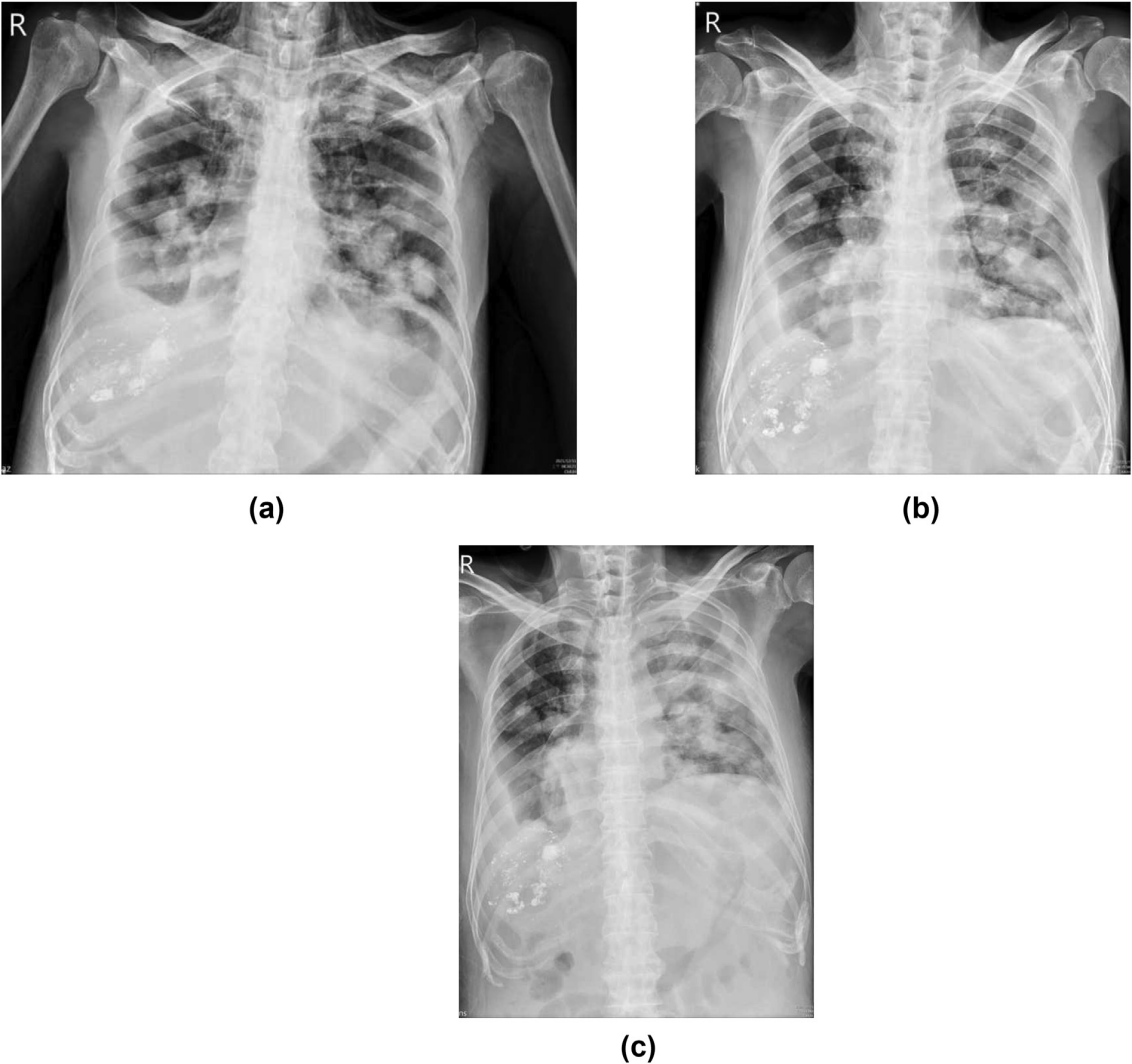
a. The anteroposterior (AP) view of the patient’s chest X-ray film on 31 December 2021. Before using pembrolizumab, multiple metastatic tumors were in both lungs, and the border of each tumor was sharp and distinct. Bilateral pleural effusions were noted. Besides, the patient also had subcutaneous emphysema in the lower neck and upper chest wall, a complication of radiotherapy to his mediastinal lymphadenopathy in Dec 2021. b. AP view of the patient’s chest X-ray film on 7 January 2022. The border of metastatic tumors became blurred with increasing infiltration. This phenomenon might be due to the clearance of HCC by T cells and inducing inflammatory reactions. c. AP view of the patient’s chest X-ray film on 14 January 2022. The infiltration area, especially in the left lung, became more prominent than that one week ago. There was no apparent ground glass pattern in the right upper lobe. This phenomenon might be because there were no evident metastatic nodules in this area. Ten days later, left tension pneumothorax developed. This event might result from the necrosis of metastatic tumors in the left lung.
